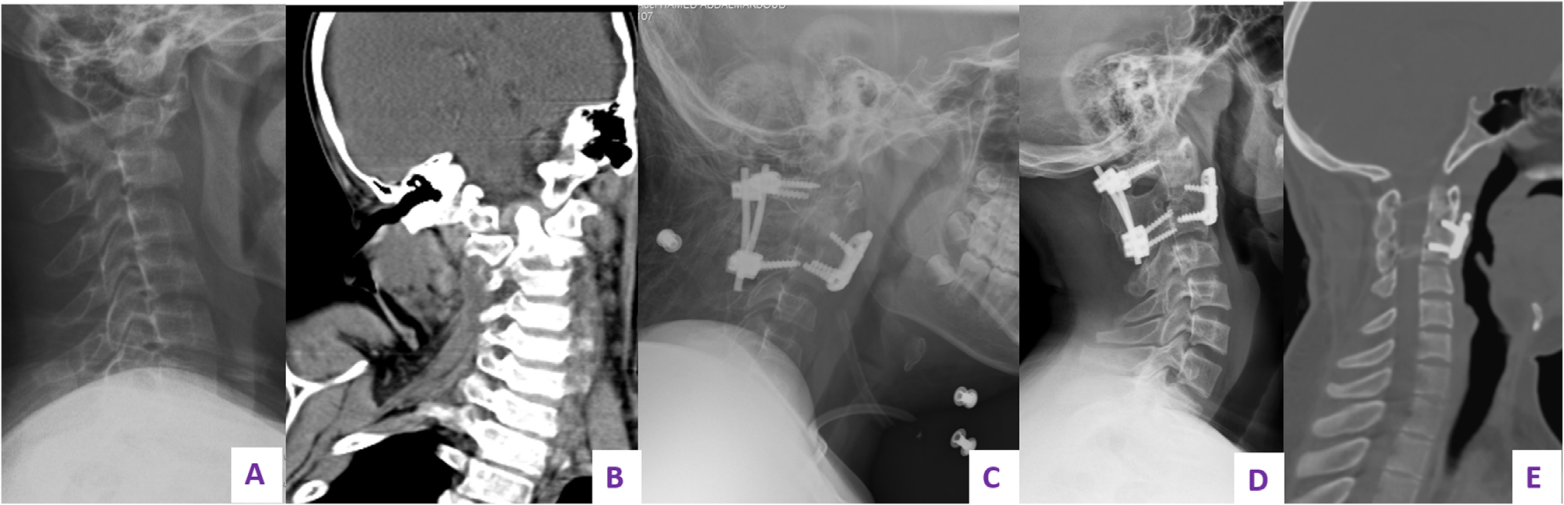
Plain radiograph of cervical spine of a 12-year-old boy diagnosed with LCH showing destruction and atlanto-axial instability, surgery was mandated to avoid neurological affection. (A) Preoperative plain radiograph showing C1–C2 instability. (B) CT scan showing the instability and lateral tilt of C1 over C2. (C) Postoperative radiograph showing C1–C2 fixation. (D) Follow-up radiograph after 1 year. (E) Follow-up CT scan after 5 years showing complete fusion of C1–C2.
